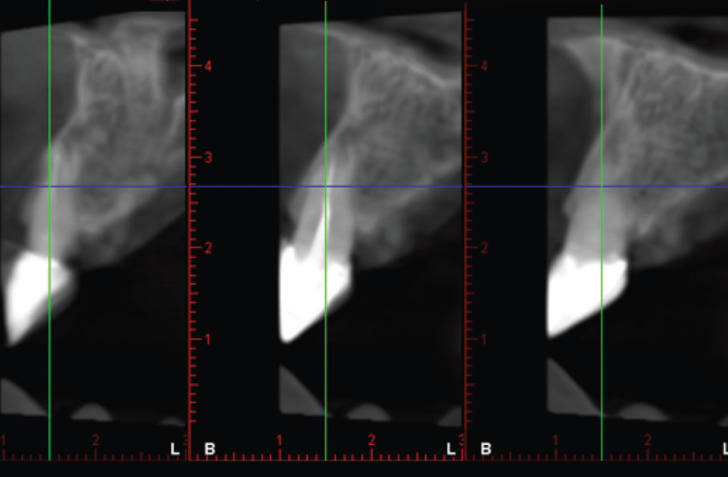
Cone Beam Computed Tomography (CBCT) of restored tooth showing the presence of cortical bone on the vestibular and palatine aspects.
